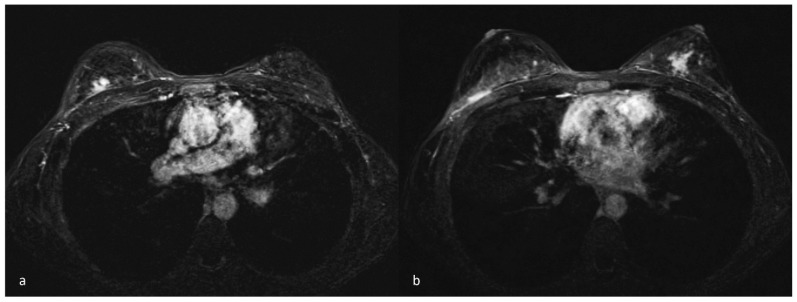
MRI subtraction images of a 38-year-old woman with a DMP diagnosis following a core needle biopsy show two inhomogeneous mass-like enhancements with a persistent kinetic pattern, irregular shapes, and spiculated margins in the upper outer quadrant of the right breast (a) and in the retroareolar areas of the left breast (b).
